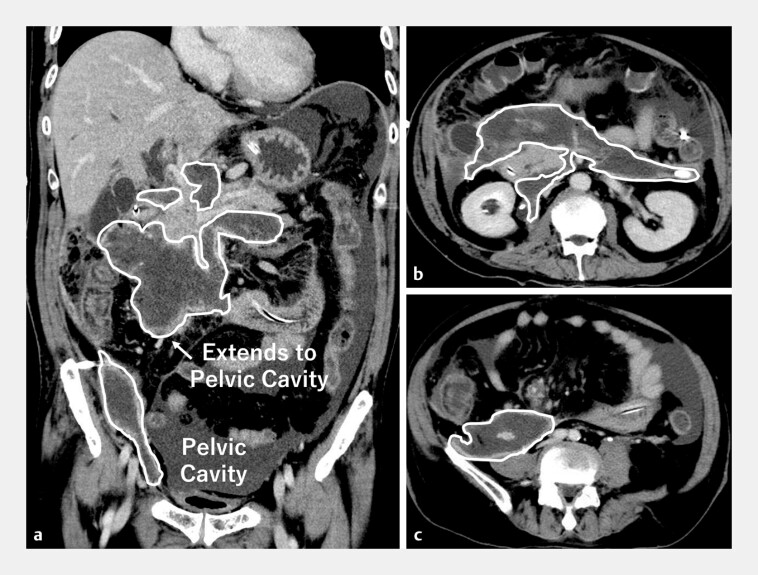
Computed tomographic images obtained before the initiation of percutaneous endoscopic
